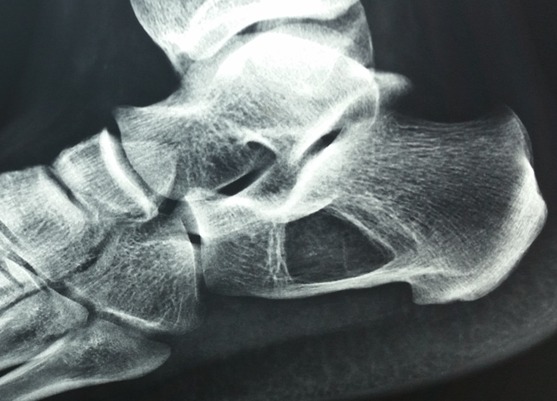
Radiographie de profil de la cheville montrant la lacune calcanéenne
